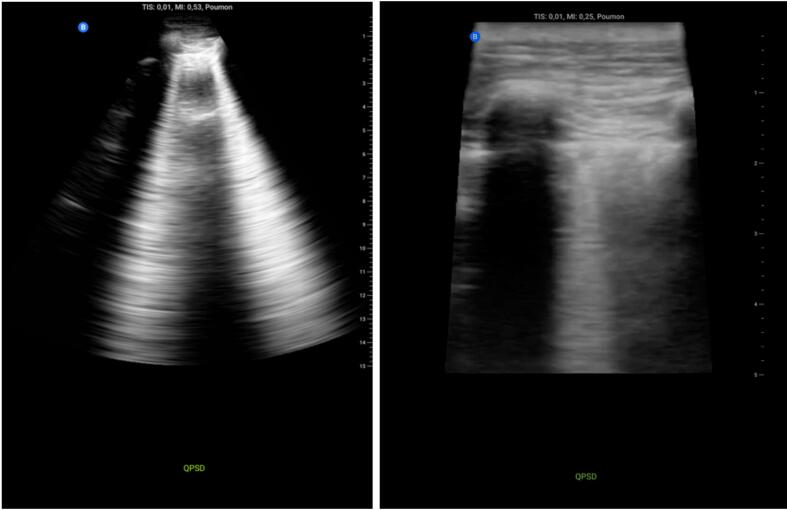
Left; sonographic image in lung mode at 15 cm depth (as a convex or phase-array probe) of two B-lines, note that the detailed aspect of the pleural line is invisible in this view Right; same quadrant viewed in lung mode at 5 cm depth (as a linear probe). Details of the aspect of the pleural line become apparent and we note an irregular pleural line with a hypo-echoic subpleural nodule.
